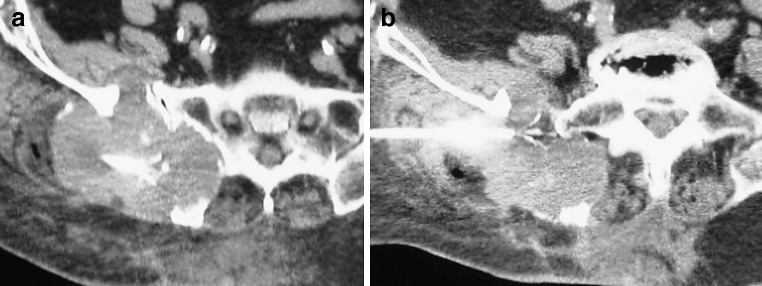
Computed tomography scan images at the level of the sacrum show a, b two different electrode placements within a soft tissue mass involving the sacrum and right iliac bone (metastasis from thyroid cancer). Before the radiofrequency session, the average pain score was 7. During the first 24 h after radiofrequency, it was 4 and 1, 4, and 8 weeks later the average pain scores were 3, 2 and 1 respectively
